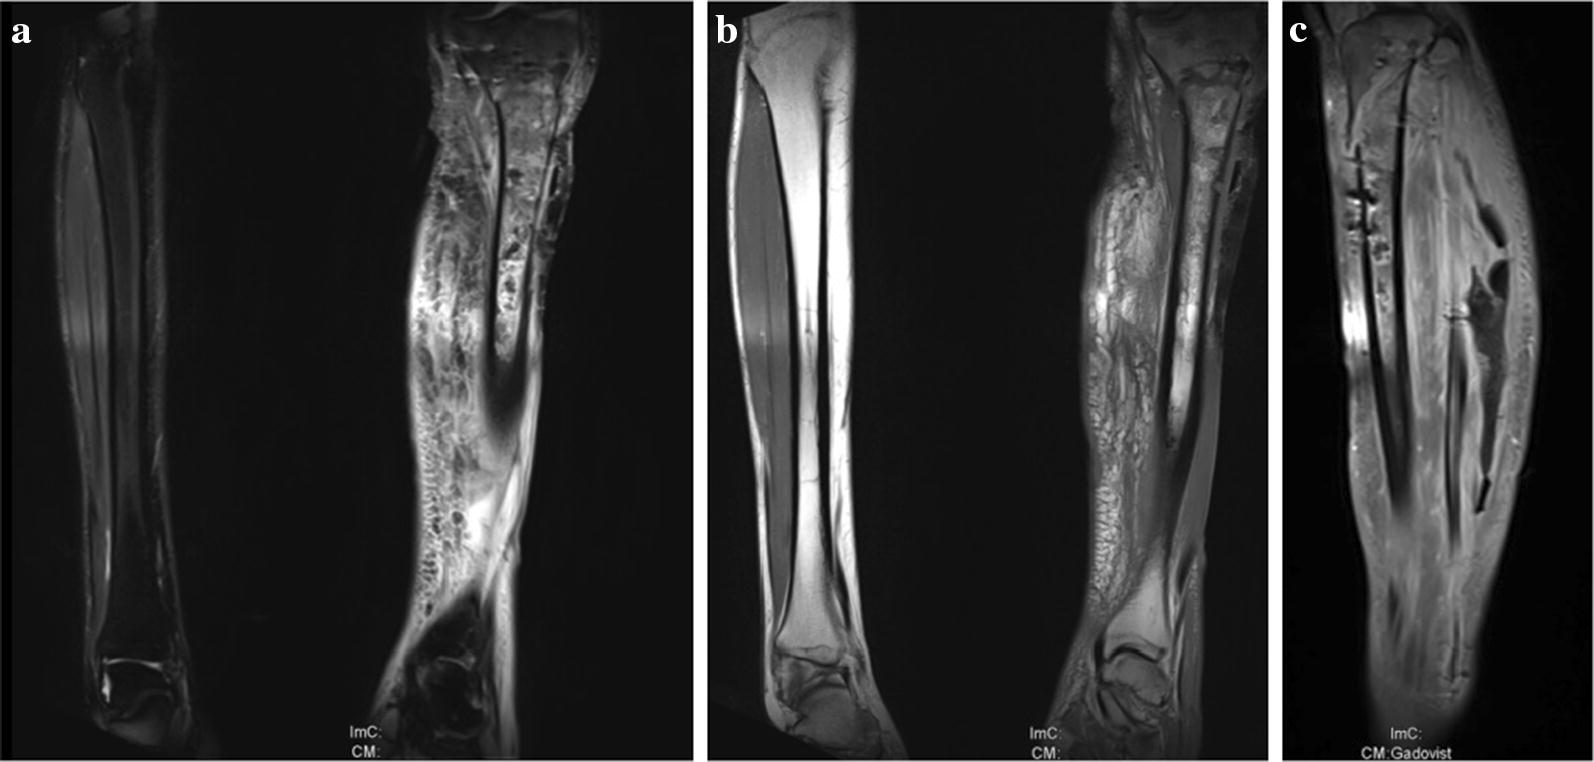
MRI scan at first admission showing septic pseudarthrosis, osteomyelitis and soft tissue abscess STIR/T1. a MRI coronary plane STIR sequence, b MRI coronary plane T1 sequence and c MRI sagittal plane STIR sequence
